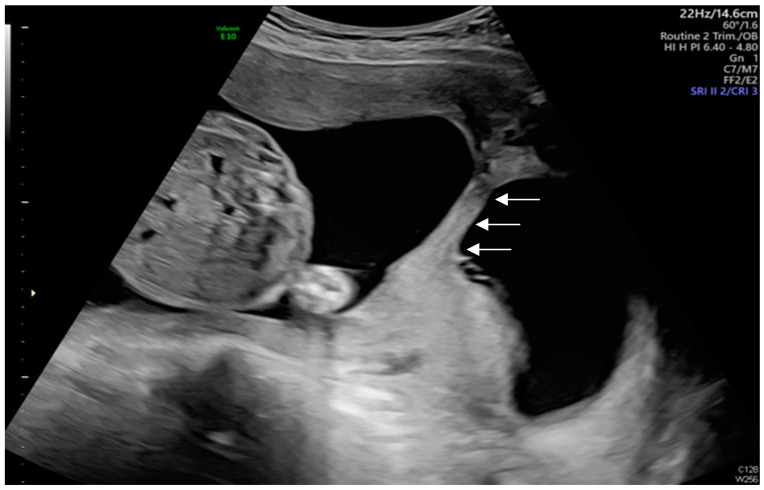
When the ultrasound probe was moved to the right side of the uterus, the adhesion-induced septa were visualized perpendicular to the anterior and posterior uterine walls, without inlet (As shown by the white arrows).
